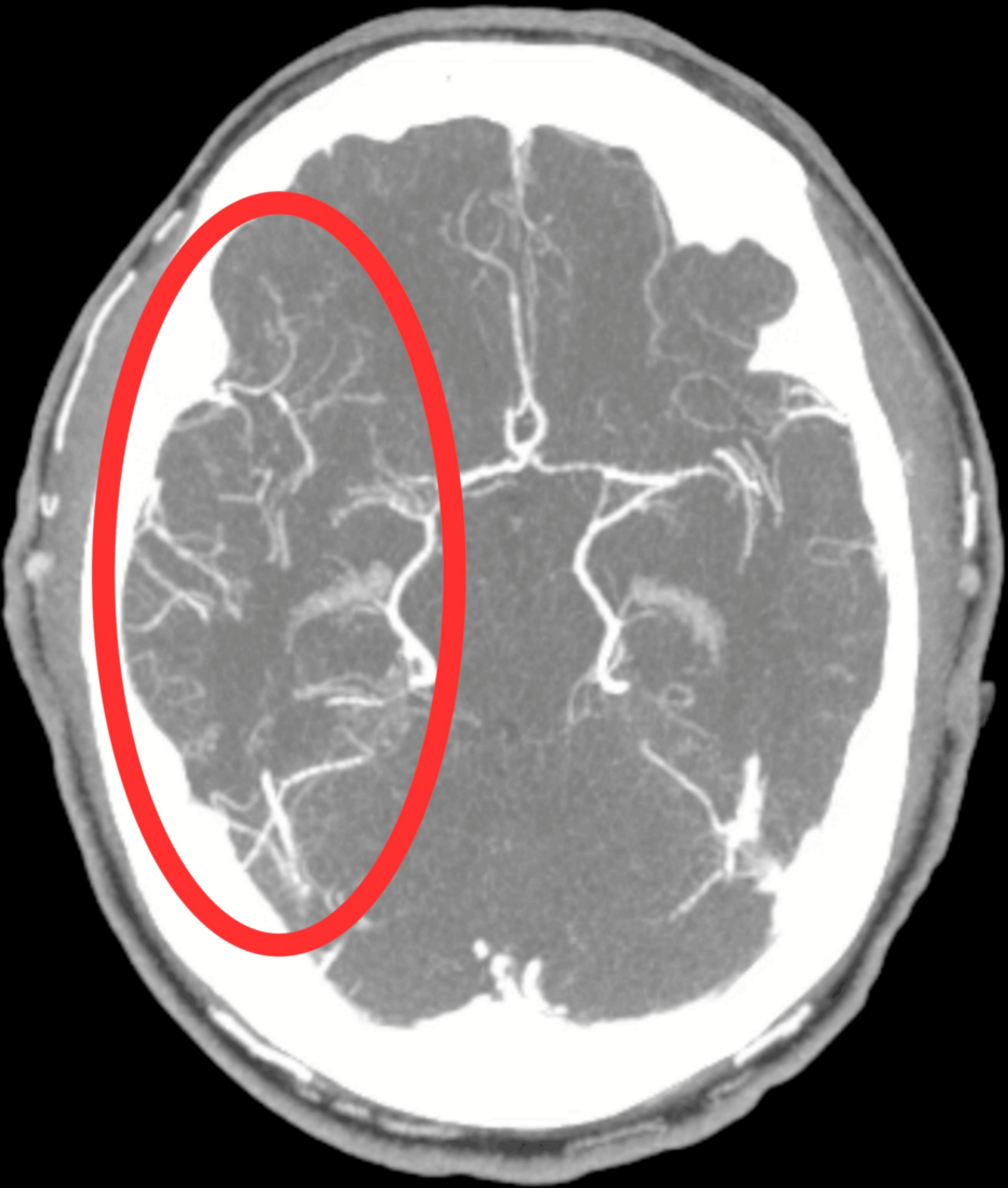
Axial view of CTA demonstrating occlusion of the right M1 segment of the middle cerebral artery (red oval), along with extensive collateral vessel formation at the base of the brain. CTA: Computed tomography angiography
